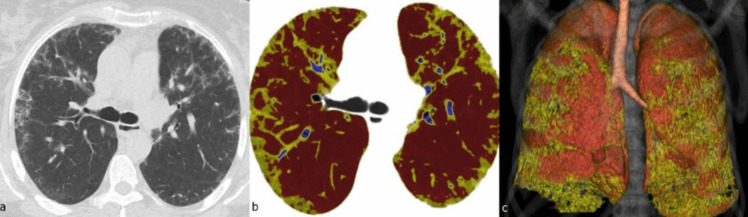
A case of quantitative imaging (patient with HRCT images shown in Fig. 1). In the case study, involvement in the form of peripherally located patchy thin reticulation is observed in the axial HRCT section passing through the carina level (a). In the quantitative analysis of the cross-section passing through the same level, the threshold values between − 700 and − 200 HU, which correspond to the regions held, are represented in yellow (b). Three-dimensional and color quantitative analysis of the same patient is monitored (c)
